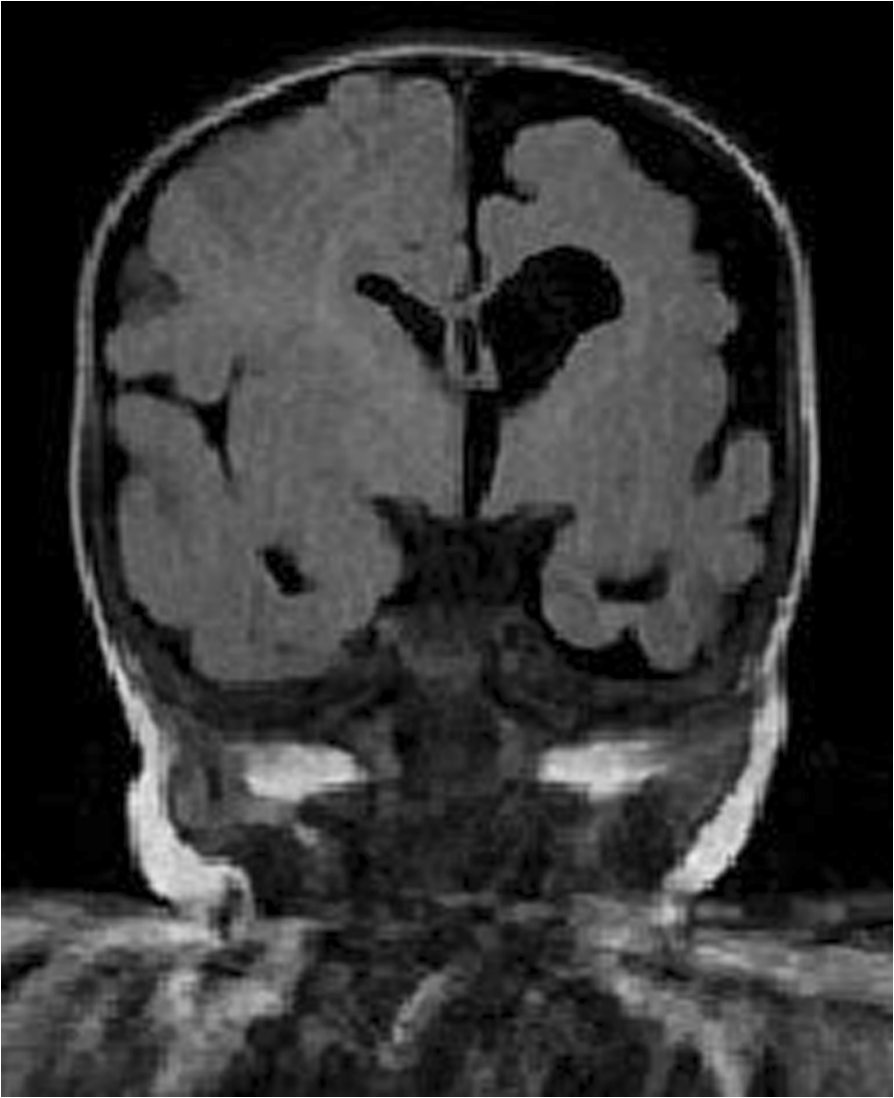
Two-month-old patient. Brain MRI coronal FLAIR image shows atrophy of the entire left hemisphere with compensatory ipsilateral midline structure shifting.
